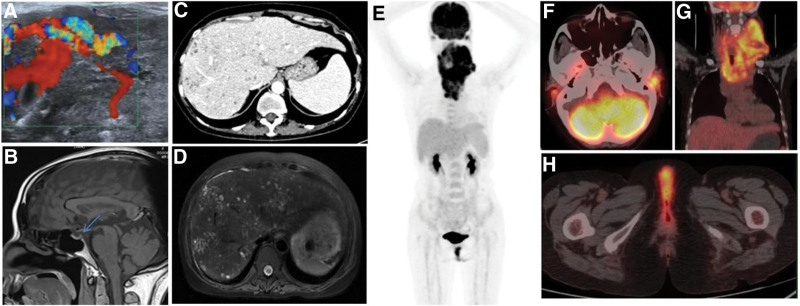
(A) The left thyroid lobe was enlarged and blood flow was abundant as shown by CDFI. (B) In the sagittal T1WI MRI view, the signal from the posterior pituitary lobe was markedly reduced (blue arrow). (C) CT scan showed hepatosplenomegaly with many small round and slightly condensed shadows in the liver. Also increased venous phase with mild enhancement in the marginal area. (D) T2WI view showed multiple small round shadows with high intensity in the liver. (E) The maximum intensity projection of the 18FDG PET/CT whole-body image showed shadows in the bilateral neck, upper mediastinum, and thyroid area. Nodules and streaks with concentrated shadows in the bilateral external auditory canal and perineal area. (F) PET/CT fusion image showed increased FDG uptake of the lesion in the bilateral external auditory canals with a SUVmax of 5.8. (G) PET/CT fusion image showed diffuse thyroid enlargement with increased FDG uptake and a SUVmax of 10.3. Multiple swollen lymph nodes were visible on both sides of the neck. The left side had coalesced into a cluster with increased FDG uptake and an SUVmax of 9.3. (H) PET/CT fusion image in transverse view described increased FDG uptake in the perineum with an SUVmax of 9.9. CT = computed tomography, FDG = fluorodeoxyglucose, MRI = magnetic resonance imaging.
